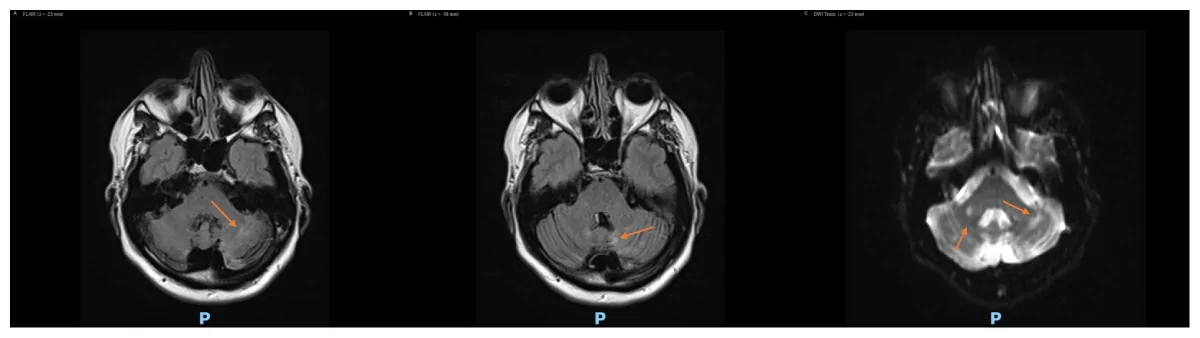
Brain MRI. (A) and (B): Axial FLAIR sequence at two infratentorial levels showing small T2/FLAIR hyperintense foci in both cerebellar hemispheres and the right middle cerebellar peduncle (red arrows), with cerebellar atrophy. (C): Diffusion-weighted imaging (DWI Trace) at the same level demonstrating absence of diffusion restriction, excluding acute ischemic etiology. Findings were stable over a 26-month interval. Images fully deidentified with written patient consent.
